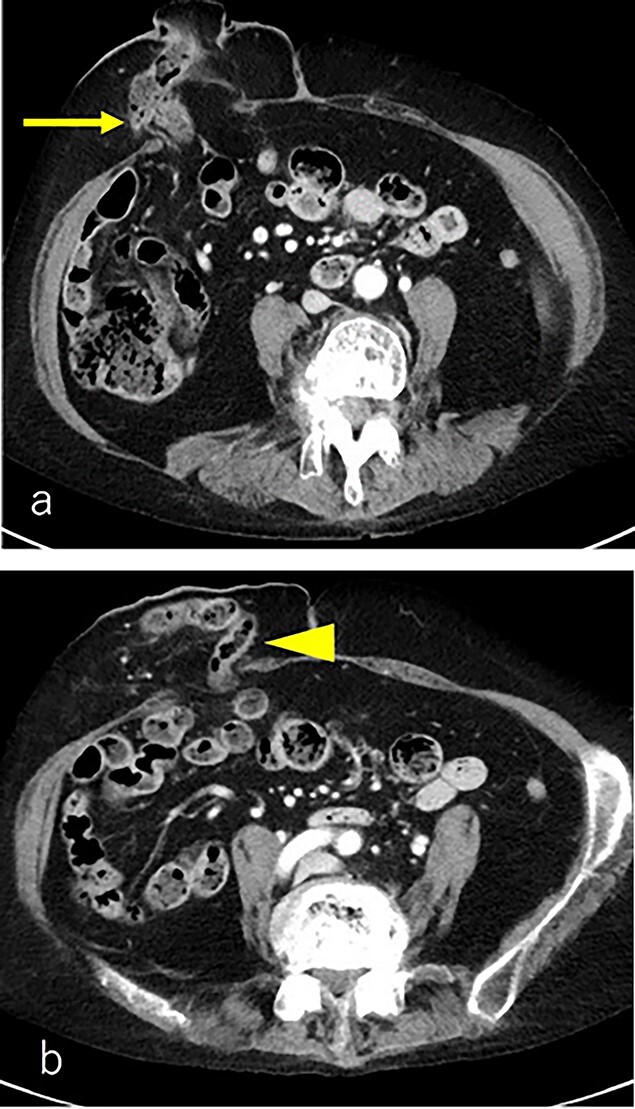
(a) CT scan revealed a prolapse of the transverse colon on the oral side (arrow); (b) CT scan revealed a prolapse of the transverse colon on the anal side (triangle).
